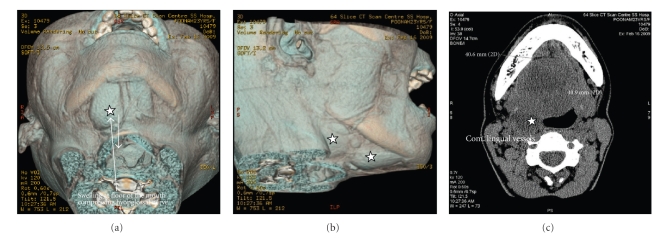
(a) and (b) CT scan of the soft tissue showing a diffused swelling involving the carotid and digastric triangle on the right side (∗). (c) The swelling extending into the retropharyngeal space on the right side (∗).
